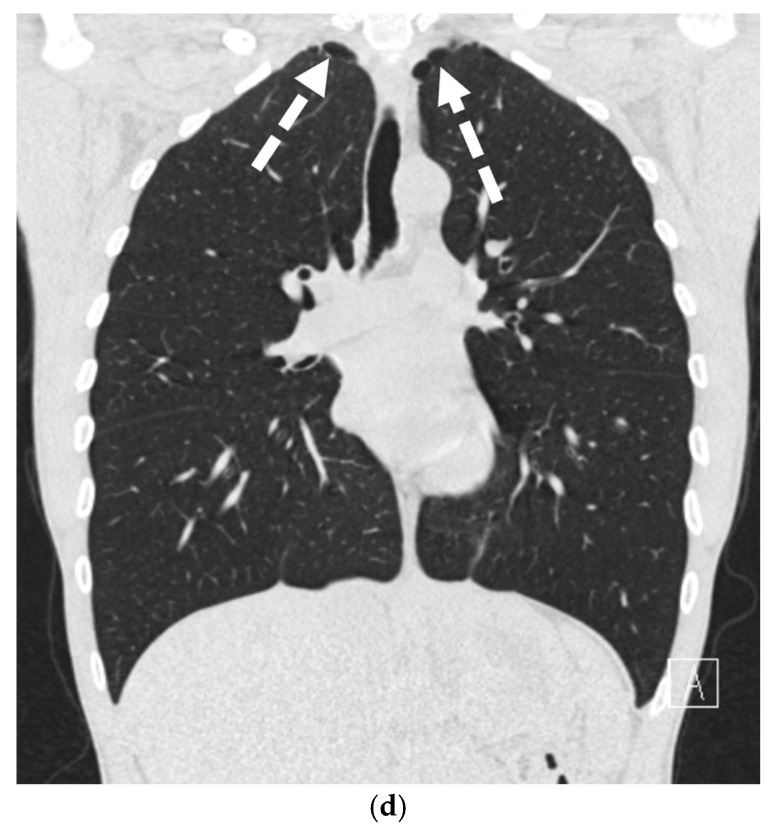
31-year-old male with a history of vaping nicotine-containing substances presents with chest pain. The frontal chest radiograph (a) is unremarkable. Axial and coronal CT images in lung window (b–d) demonstrate small right pneumothorax (open black arrow) as well as biapical paraseptal emphysema and blebs/bullae (dashed white arrows).
